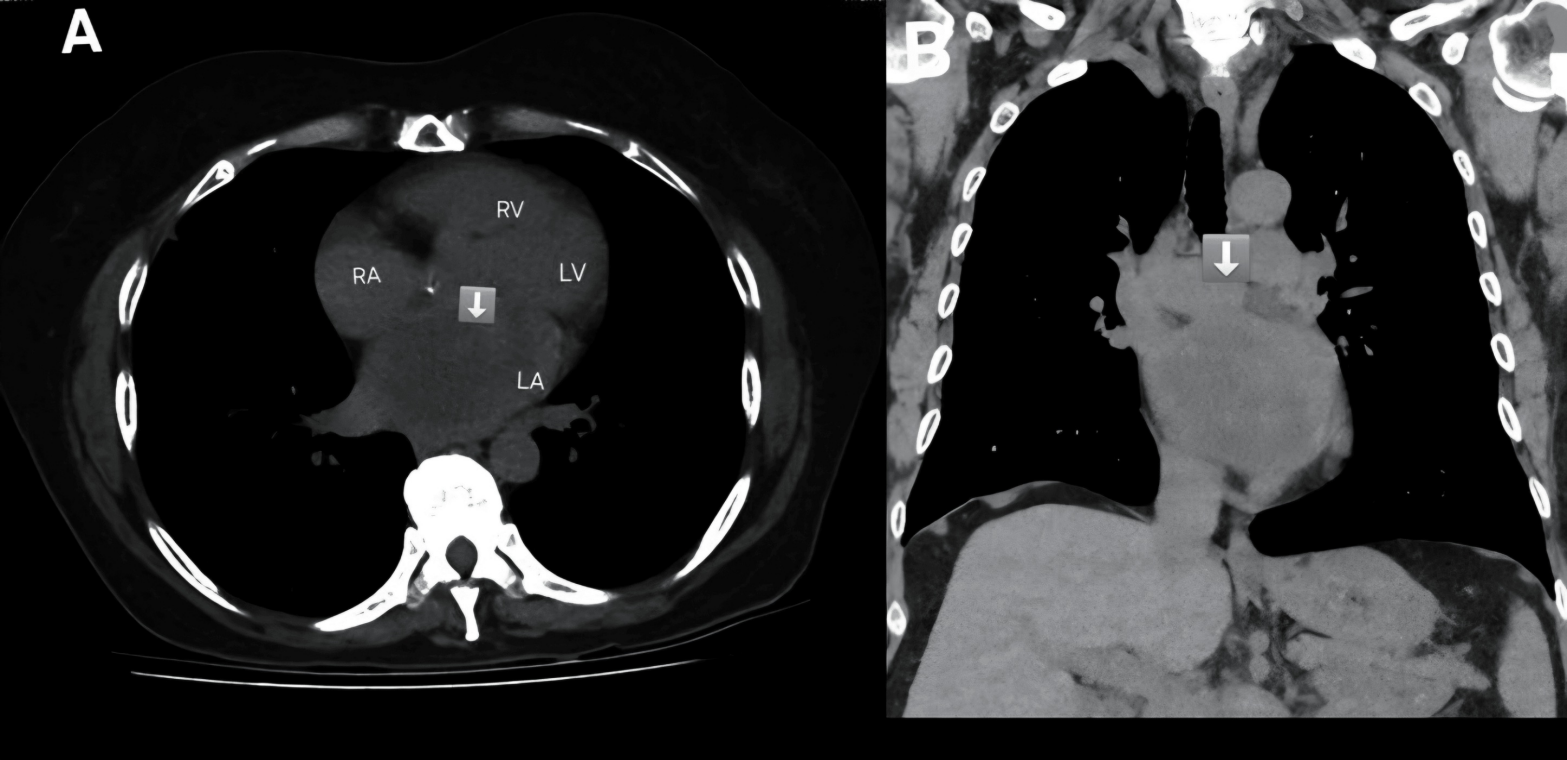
Bimodal CT assessment of left atrial myxoma (A) Axial CT view showing a solid tissue mass, concurrent with a hypodense lesion in the left atrium. (B) Coronal CT view showing a large hypodense lesion in the left atrium CT: computed tomography
